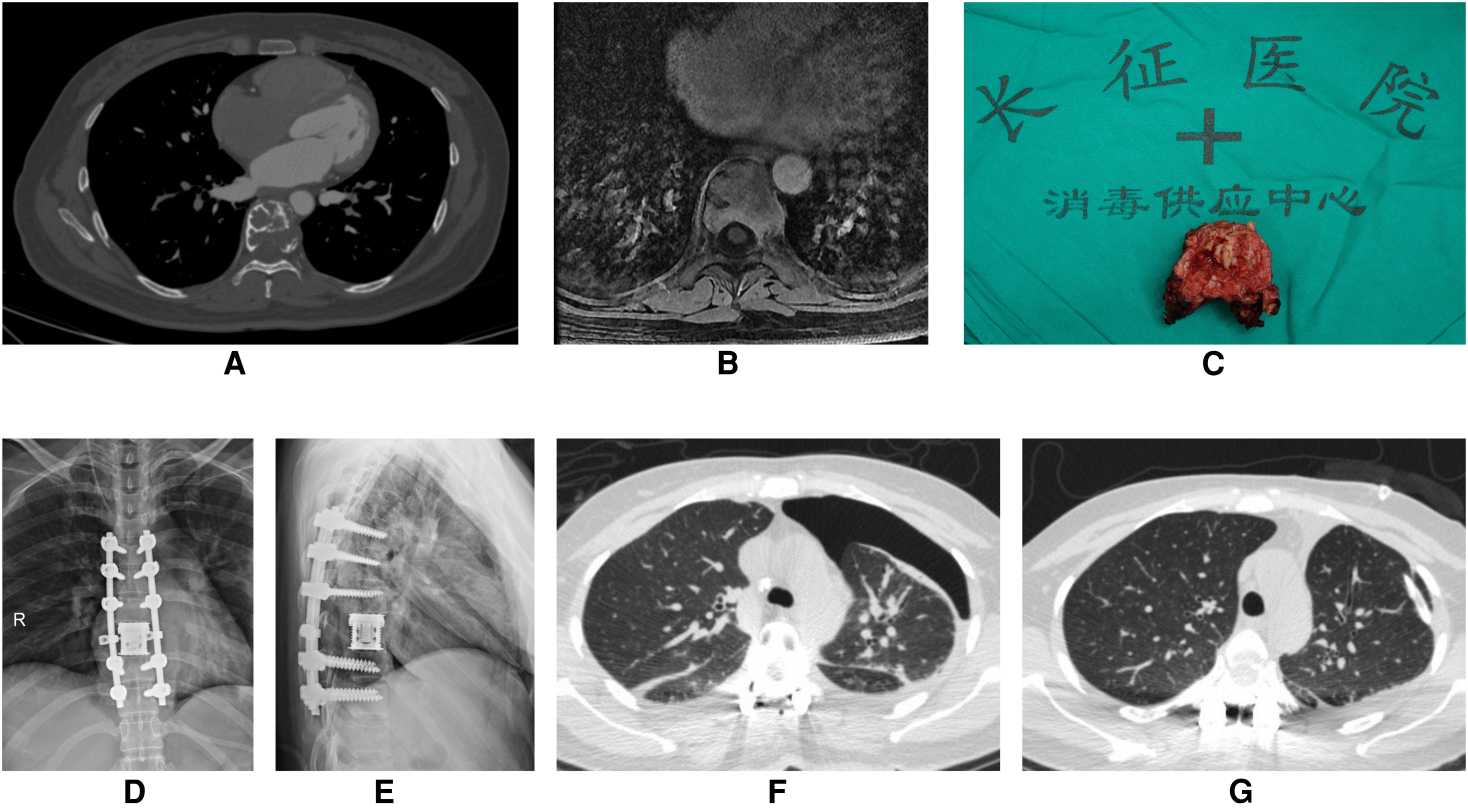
A 43-year-old woman underwent T8 *en bloc* spondylectomy for giant cell tumor of bone through the posterior approach. (A) Preoperative enhanced CT showed that T8 vertebral body had osteolytic bone destruction and a soft tissue mass. There were bone ridges and sclerotic rims in the bone destruction area. (B) Preoperative enhanced MRI showed bone destruction of T8 vertebral body with heterogeneous enhancement. (C) En bloc resection of giant cell tumor of thoracic spine (T8). (D,E) Anteroposterior and lateral x-ray of the thoracic spine after surgery. (F) On the first postoperative day, Chest CT showed severe left pneumothorax. (G) After left chest closed drainage, reexamination of chest CT showed the left lung was re-expanded well.
